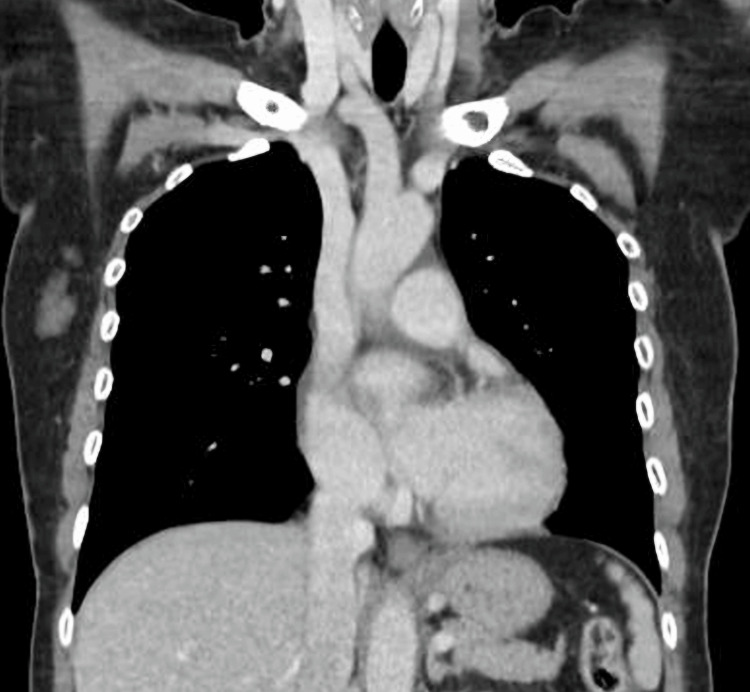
Coronal CT thorax demonstrating complete resolution of pleural and pericardial effusions The effusions are similar to those observed in Figure 2 CT: computed tomography
